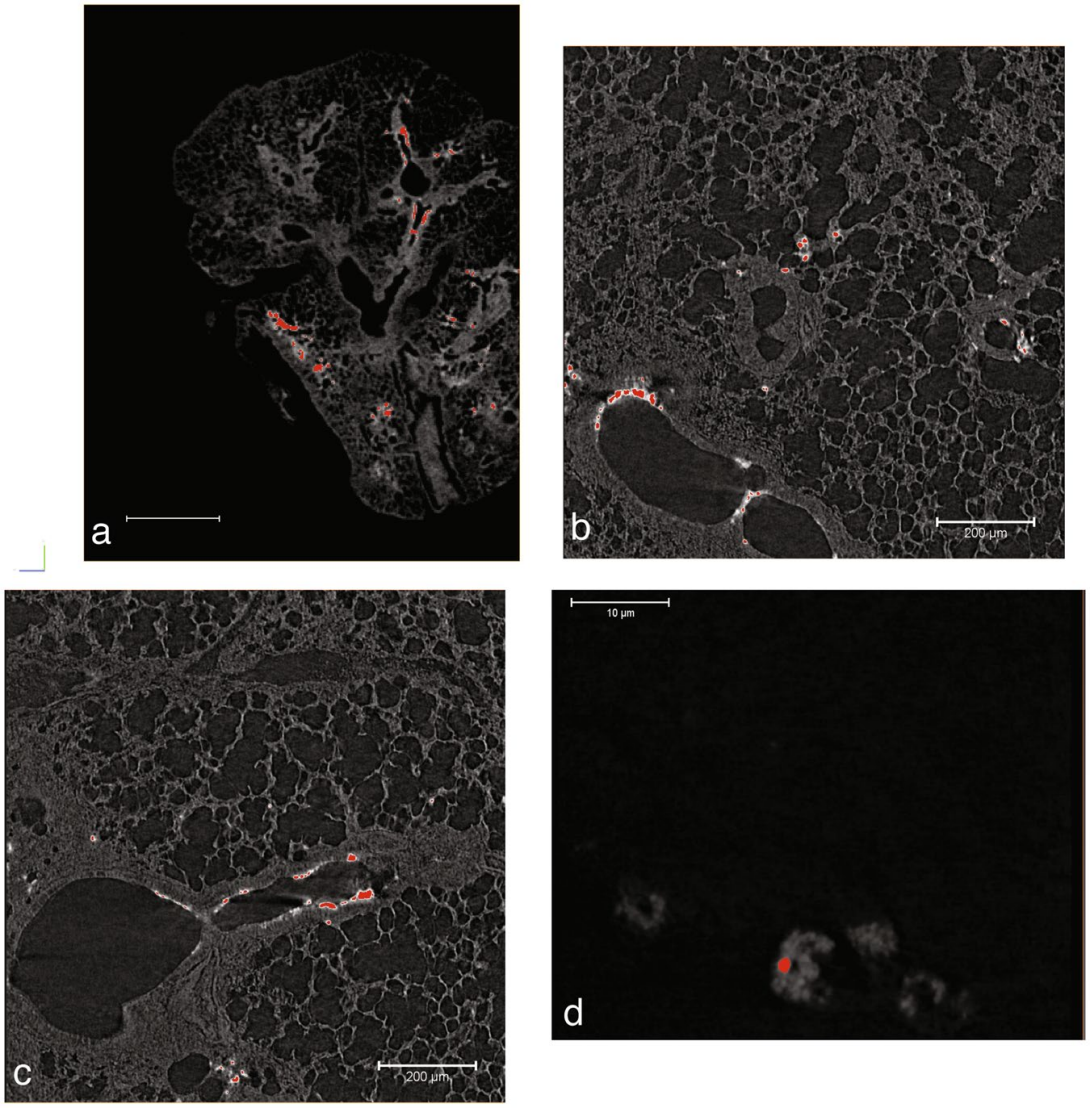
2D slices virtually extracted from reconstructed 3D images, a grayscale colormap is used. Segmented pixels are colored in red. (a) LFOV scan, 1 px = 14.32 µm, scale bar = 1000 µm (b,c) HRes scan, 1 px = 1.09 µm, scale bar = 200 µm (d) nano-CT scan, 1 px = 63.5 nm, scale bar = 10 µm.
